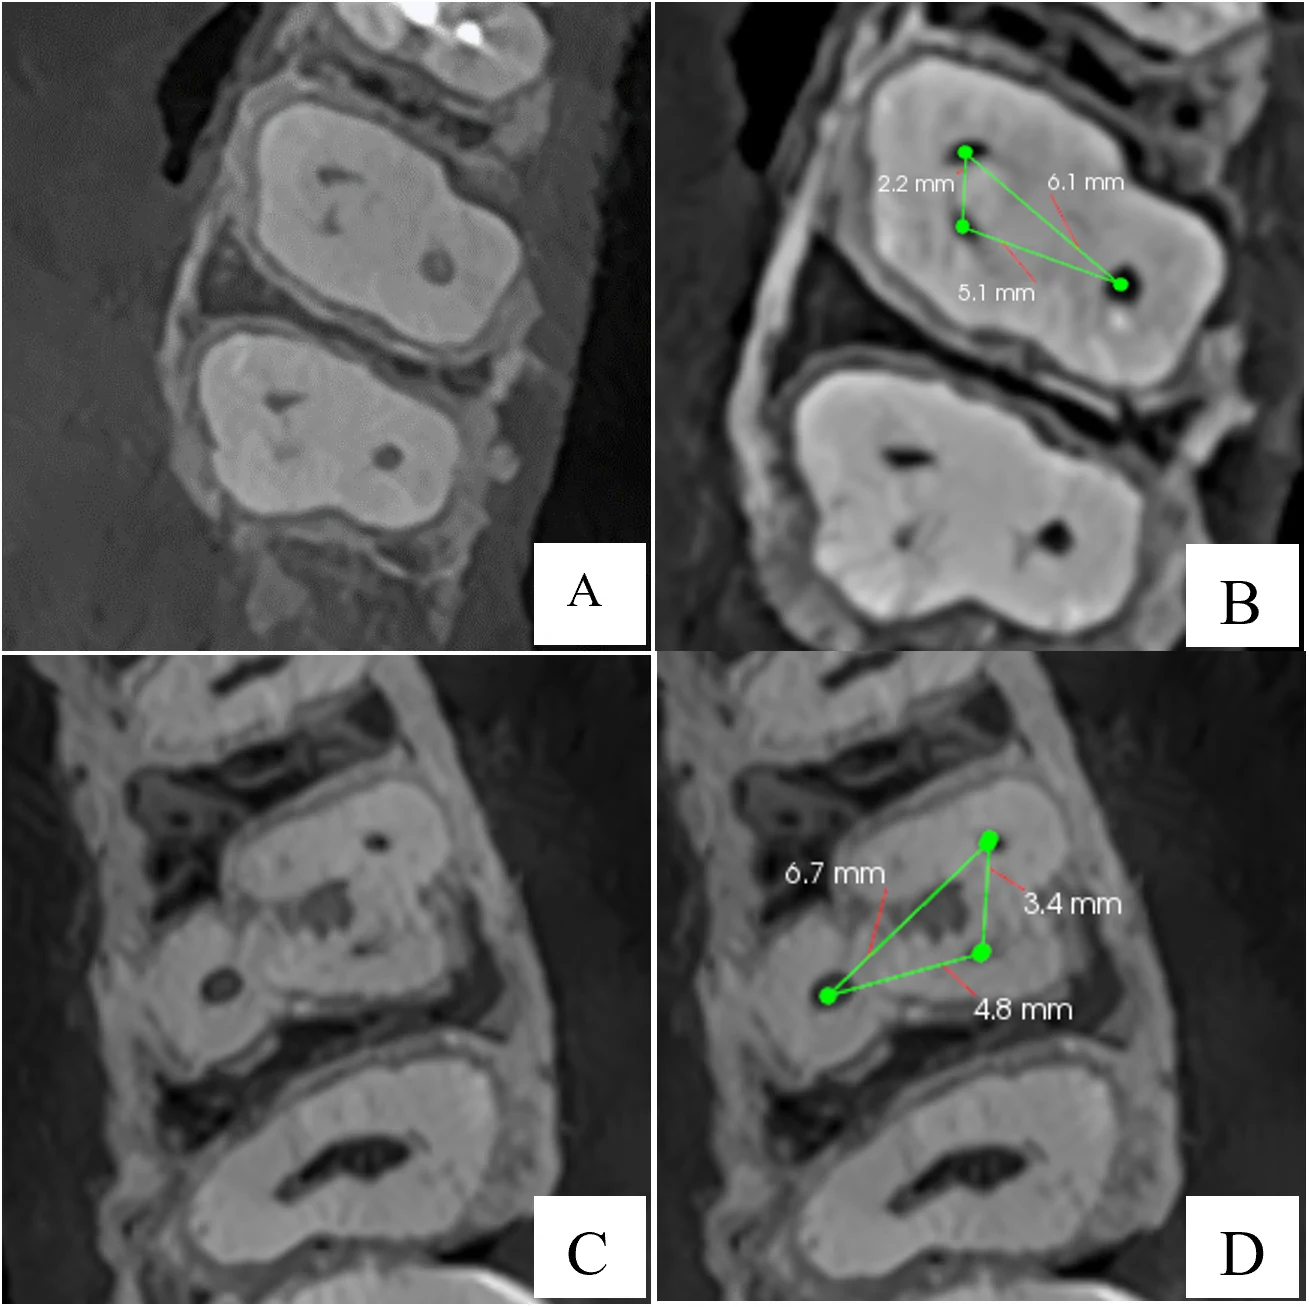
Axial CBCT slices at the level of the pulpal floor showing the initial identification of canal orifices of the MFMs: A: No MB2 canal; B: The interorifice distance measurements between MB, DB, and P canal in absence of MB2 canal; C: with MB2 canal (red arrow); D: The interorifice distance measurements between MB, DB, and P canal in presence of MB2 canal. Note: green points indicate the center of each canal orifice, and the connecting lines represent the linear distances (in millimeters) between canals.
